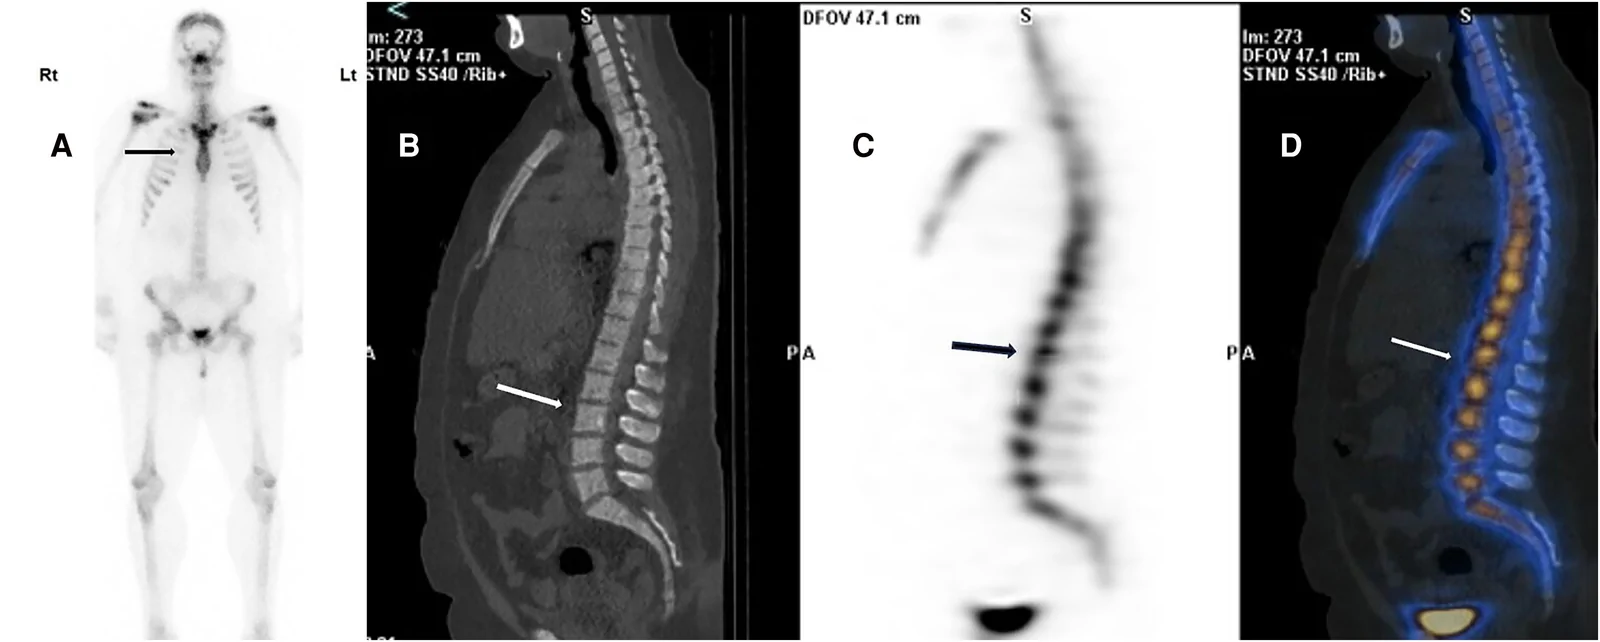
Panel A: neck-tie sign on bone scintigraphy. Panel B-D: diffuse crisp radiotracer uptake throughout the spine on a nuclear medicine whole body bone single-photon emission CT (SPECT)/CT scan.
